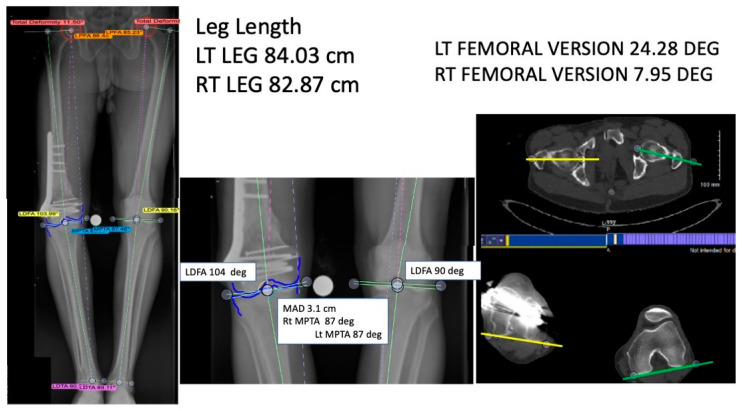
Radiographic and computed tomography assessment of femoral malunion. The left image displays a full-length standing radiographs and CT scans demonstrating deformity analysis in a patient with femoral malunion. In the middle image, mechanical axis deviation (MAD), lateral distal femoral angle (LDFA), and medial proximal tibial angle (MPTA) are measured to evaluate coronal alignment and leg length discrepancy (left leg: 84.03 cm; right leg: 82.87 cm). The Axial CT images on the right show femoral version differences (left 24.28°, right 7.95°), highlighting rotational malalignment. These imaging modalities assist in quantifying angular, rotational, and length deformities prior to corrective planning.
